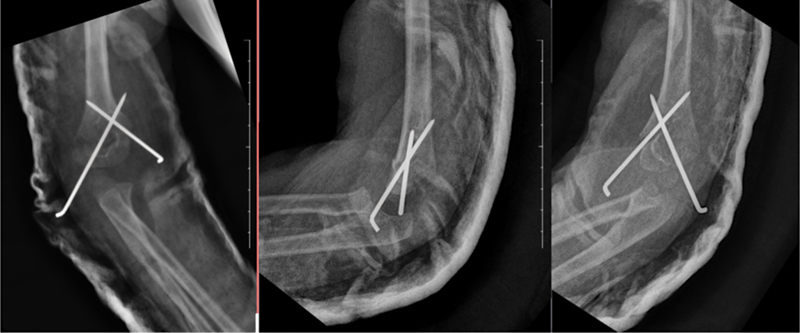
Anteroposterior and lateral oblique radiographs of the distal humerus performed immediately after surgery, demonstrating an acceptable reduction but inadequate fixation, as one of the wires does not fixate the distal fragment.
